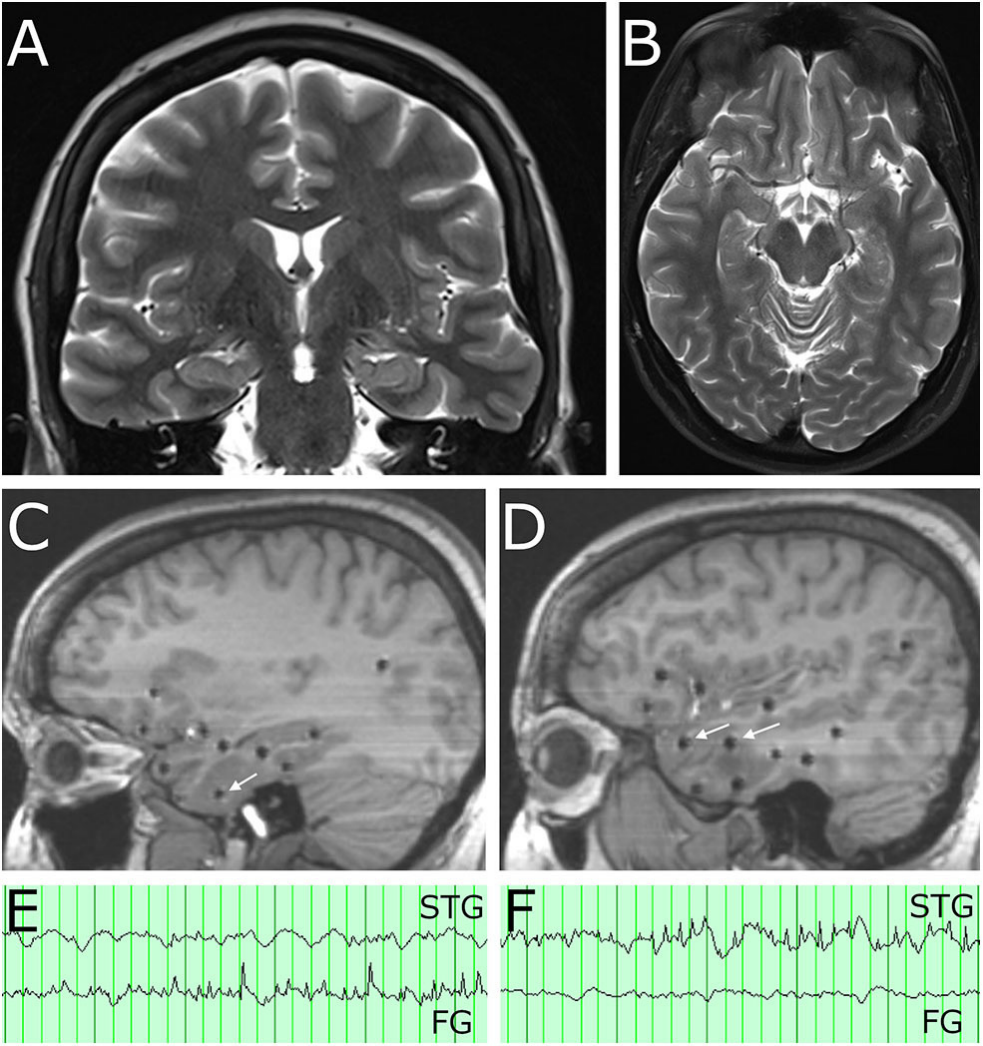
Pre-operative imaging demonstrates no abnormality on MRI and location of SEEG electrode contacts involved in the seizure network. (A) Coronal T2 MRI and (B) axial T2 MRI show normal appearance of the temporal lobes bilaterally. (C) Sagittal T1 MRI at the level of the mesial temporal lobe. White arrow indicates SEEG electrode contact in the fusiform gyrus with spread pattern electrographic activity during a captured seizure. (D) Sagittal T1 MRI at level of the lateral temporal neocortex. White arrows indicate SEEG electrode contacts in the superior temporal gyrus (anterior arrow) and middle temporal gyrus (posterior arrow) involved in spread patterns during subclinical seizures. (E) A clinical seizure (characterized by behavioral arrest and oral automatism) had EEG onset with rhythmic theta spiking, maximal on a contact in fusiform gyrus (FG) near the basal temporal pole, which evolved in frequency and morphology and then spread to other contacts in the neocortex of the superior, middle, and inferior temporal gyrus. Note that at onset of this clinical seizure, a contact in the superior temporal gyrus (STG) was not involved. (F) A subclinical seizure recorded on the same day had an onset of rhythmic theta spiking, maximal on a contact in the superior temporal gyrus (STG) which evolved in frequency and morphology and also spread to other contacts in the neocortex of the superior, middle, and inferior temporal gyrus. Note that at the onset of this subclinical seizures a contact in the fusiform gyrus (FG) implicated as the clinical seizure onset was not involved.
